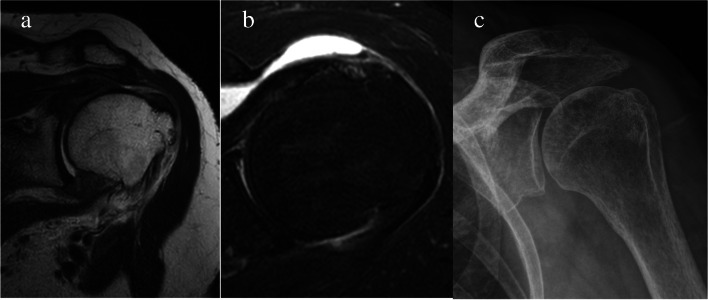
a A medium size rotator cuff tear was observed in the pre-operative magnetic resonance imaging and T2-weighted coronal view; b a nearly complete tear in the biceps long head tendon was observed in the axial view; c pre-operative plain radiography: No RDON was observed
